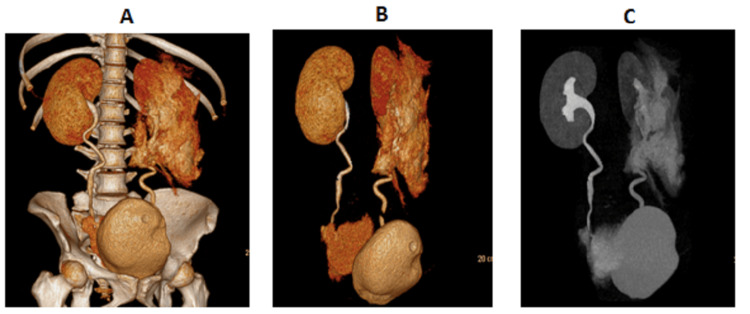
CT urogram (A,B) With contrast: post-processed 3D images further demonstrate right distal ureteric rupture with distal right ureteric extravasation of contrast leading to large pelvic urinoma that was earlier diagnosed as large pelvic collection/hematoma. (C) Without contrast: extravasation from left pelvicalyceal rupture secondary to back pressure from left distal ureteric obstruction. Right distal ureteric rupture with distal right ureteric extravasation of contrast leading to large pelvic urinoma. A CT urogram was performed to further investigate and confirm the diagnosis of ureteric injury following initial CT findings. CT, computed tomography
